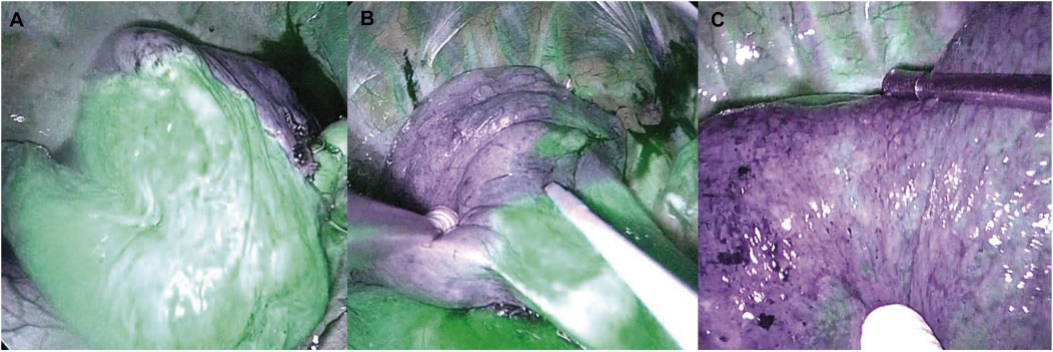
The indocyanine green demarcation score. Clear visualization without interruption (3 points, excellent) (A). Partially unclear but generally identifiable (2 points, fair) (computed tomography). Difficult to identify (1 point, poor) (C).
